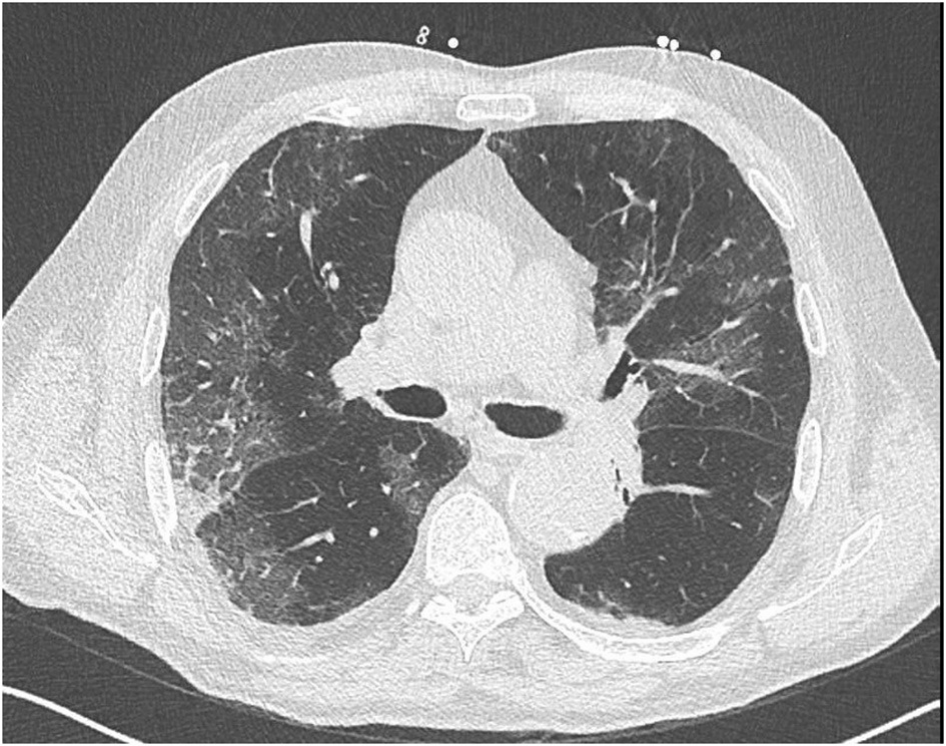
Computed tomography lung scan at baseline.
